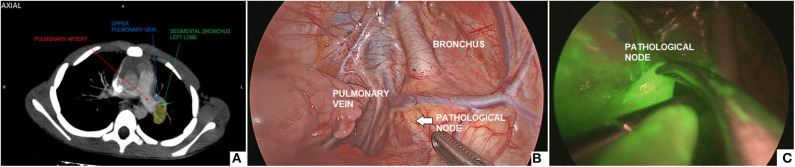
Thoracoscopic biopsy of a 2-cm hilar lymph node: pre-operative CT imaging (A), intra-operative view (B), ICG-guided NIRF imaging (C).
